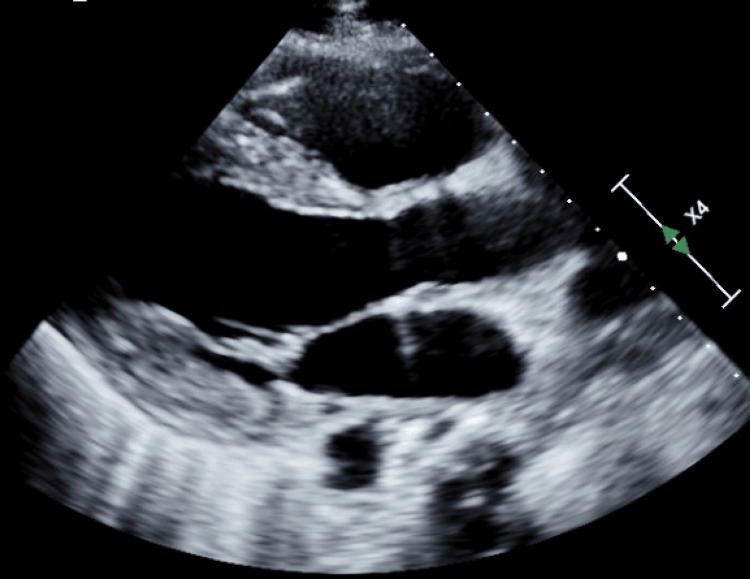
Post-procedure echocardiogram Echocardiogram taken a week following the pericardiocentesis, prior to discharge.
